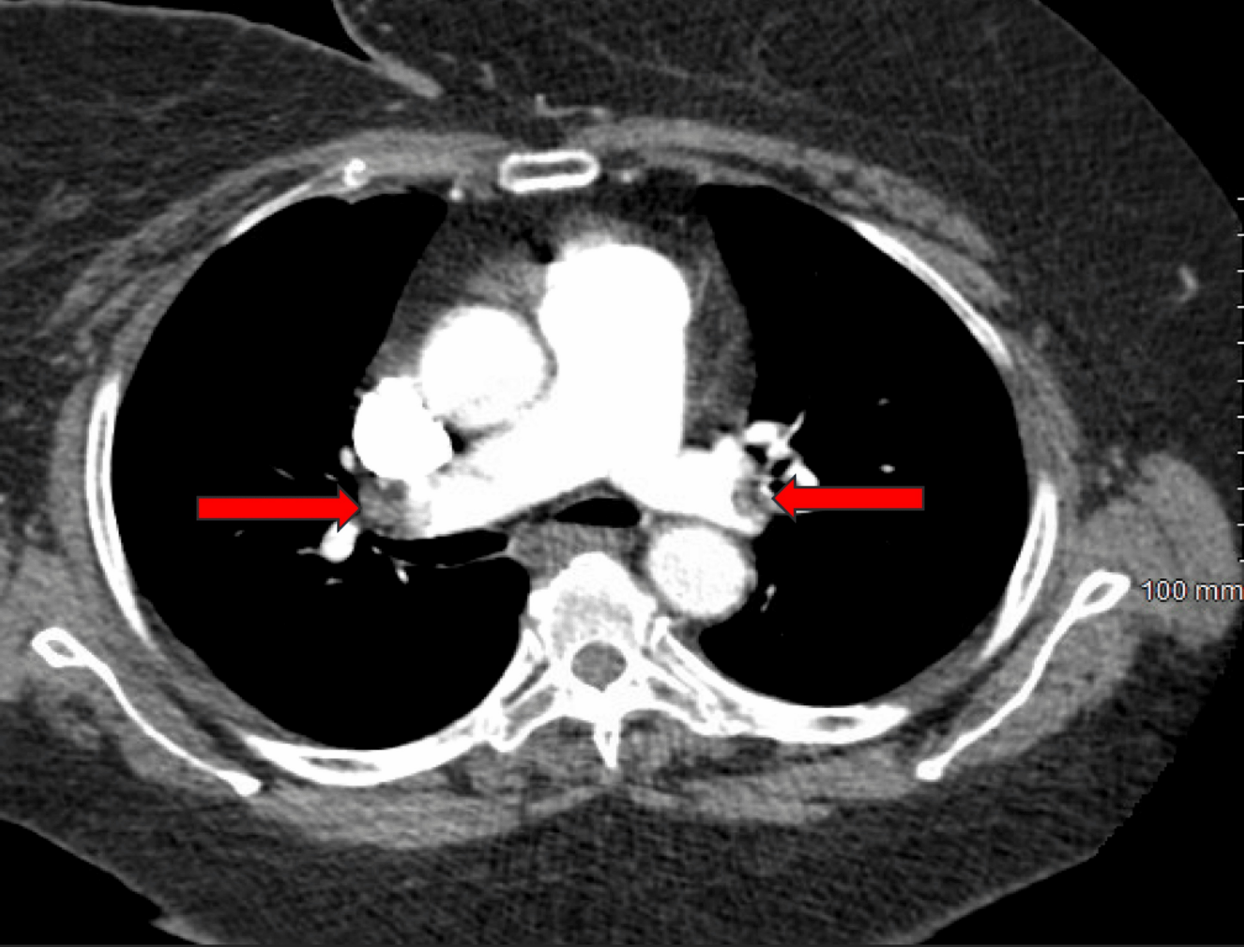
Distal thrombus in both the right and left main pulmonary arteries on CTA of the pulmonary vasculature (arrows). CTA: computed tomography angiography
